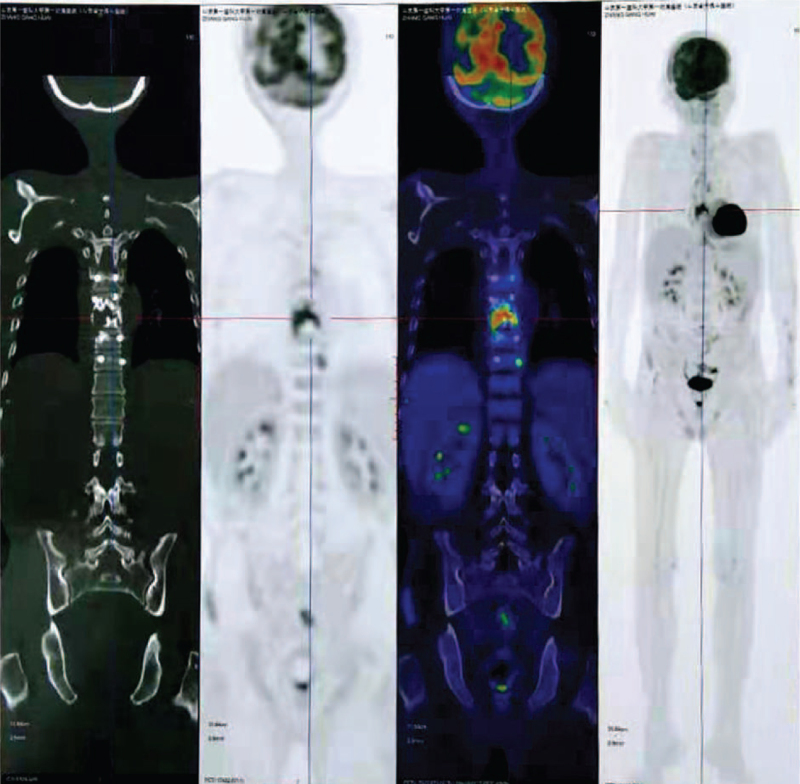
PET-CT scan showed the hypermetabolic lesion in T7 to T9.
